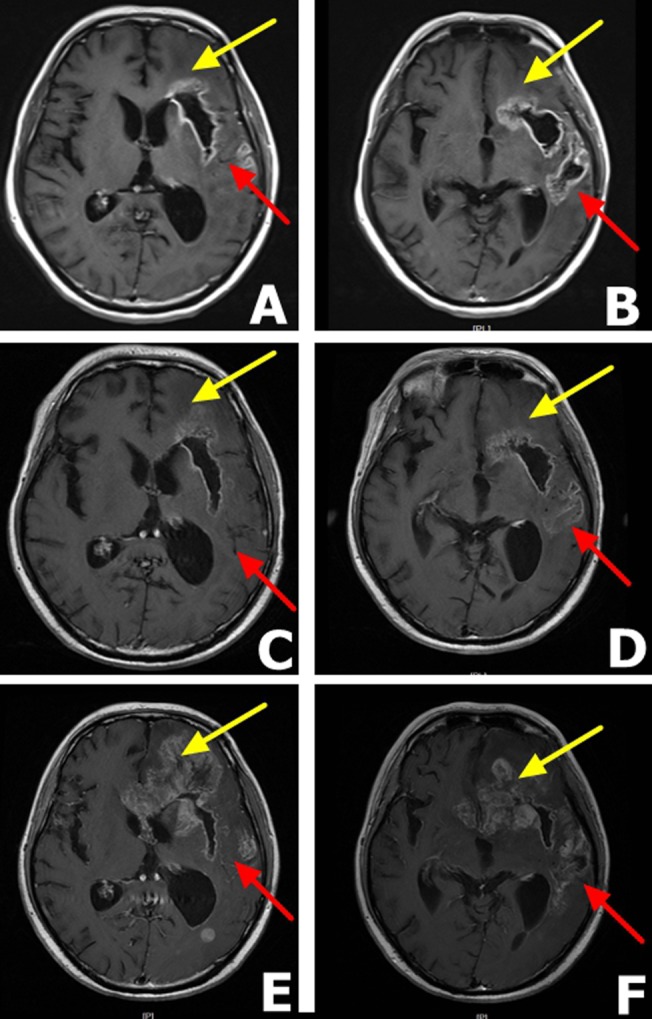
MRI findings in a male patient presenting with glioblastoma multiforme after erlotinib 150 mg therapy A. and B. MRI scans after 14 days of daily erlotinib 150 mg therapy The scans reveal tumor hollowing, lower grade edema and reduced compression of the lateral ventricles. C. and D. Three months after erlotinib 150 mg therapy, MRI scans shows that the tumor stabilized. Yellow arrow shows a stable frontal lobe while the red arrow show partial response in the temporal lobe. E. and F. Five months after erlotinib 150 mg therapy. MRI scans demonstrate the tumor relapsed for the second time with distant metastasis and the patient died two months later. The yellow arrow shows obvious metastasis in the frontal lobe and red arrow shows a stable temporal lobe.
